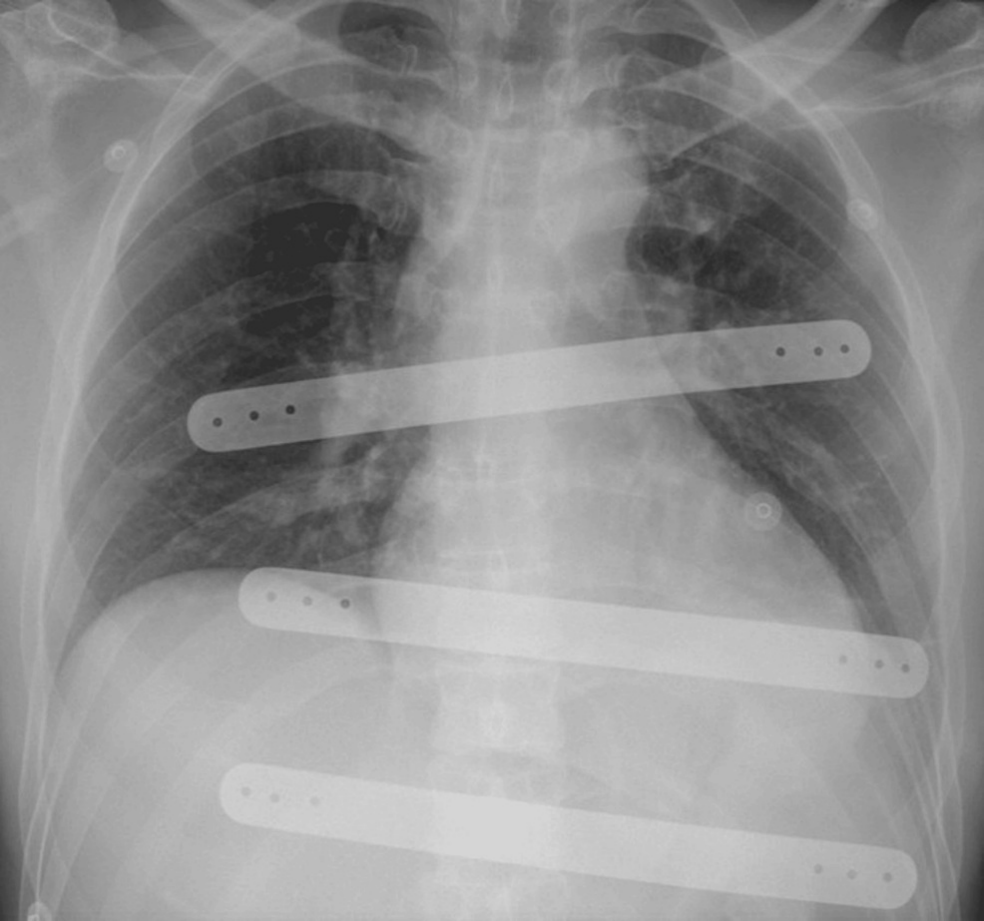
X-ray showing pectus bars used for reconstruction of the chest wall
